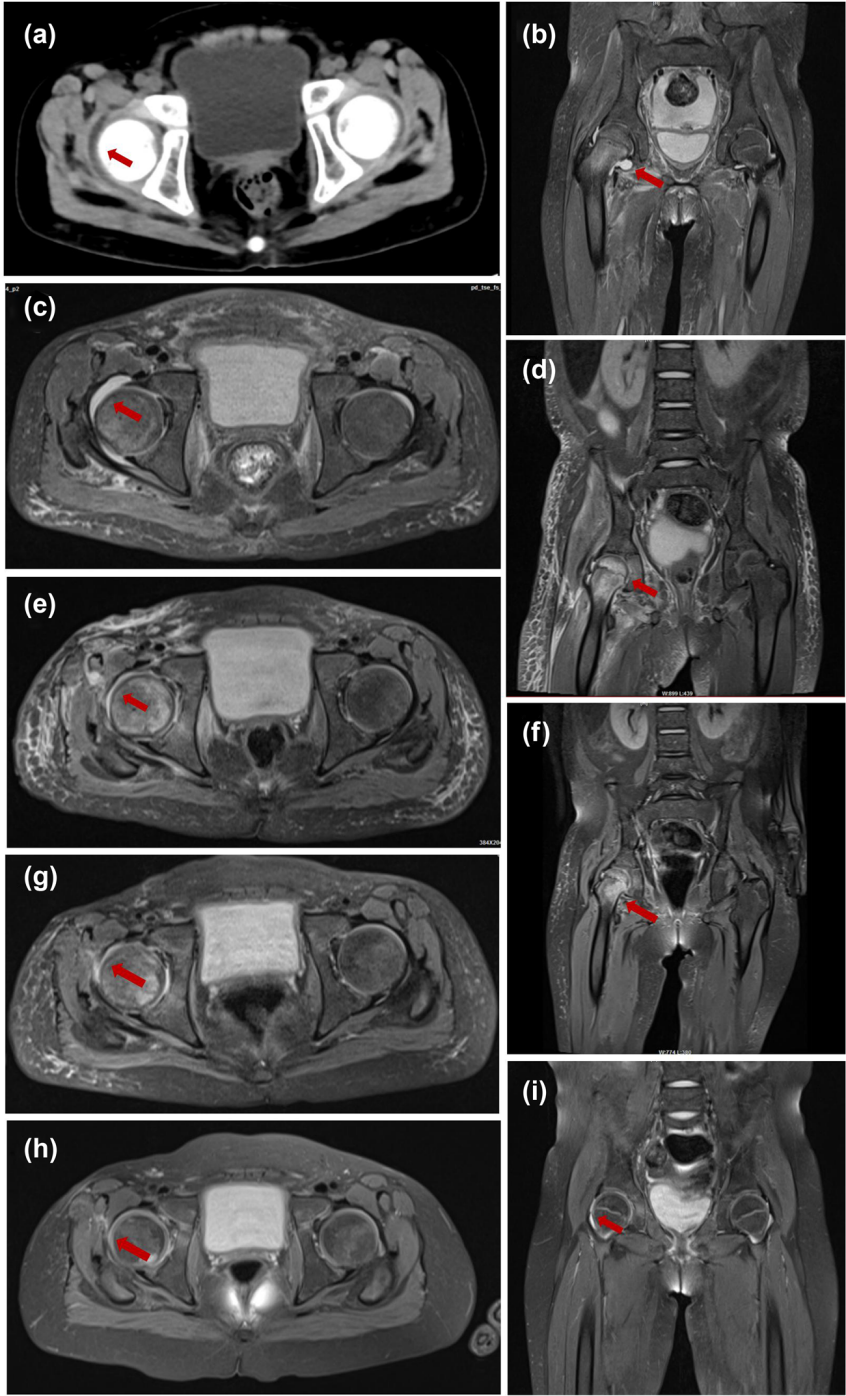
Radiograph check-up examinations. (a) Initial CT of bilateral hip joints showing aspiration of right hip joint effusion. (b) and (c) Initial MRI of bilateral hip joints showing marrow edema of right proximal femur and aspiration of right hip joint effusion. (d) and (e) Repeat MRI of bilateral hip joints on the 15th day after surgery showing a decrease in right hip capsule effusion compared to previous scans. (f) and (g) Repeat MRI of bilateral hip joints on the 26th day after surgery showing a further reduction in right hip capsule effusion and a significant reduction of soft tissue swelling. (h) and (i) Hip MRI at 1 month after discharge revealed a significant reduction in right hip capsule effusion and a marked decrease in the size of soft tissue edema. Red arrows indicate the target lesion.
